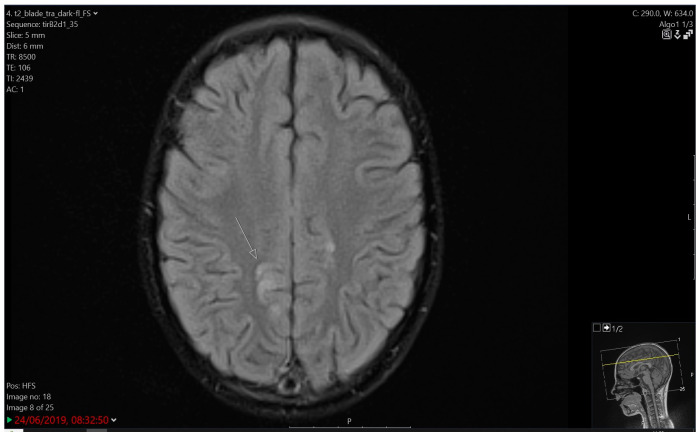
Cortical oedema correlated with the toxic effect of chemotherapy. T2/FLAIR zone of high signal intensity in the posterior segment of the cingulate gyrus on both sides, more pronounced on the right.
